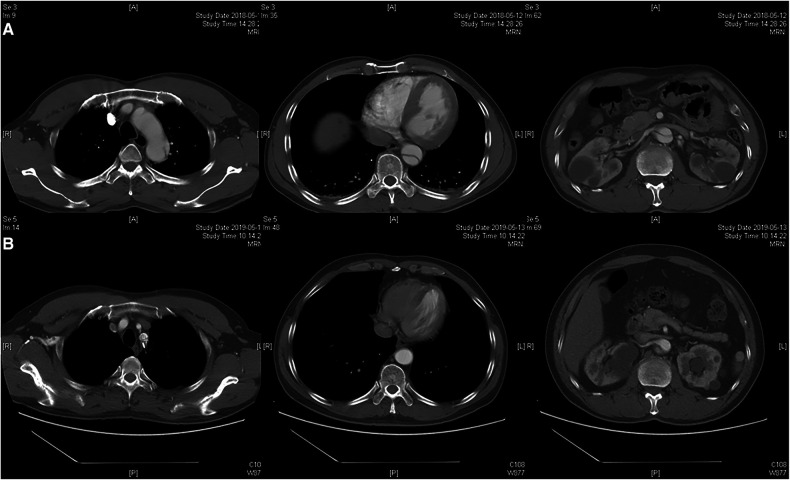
Radiographic image of chimney technique. (A) Preoperative CTA showed the dissection involving the distal aortic arch and visceral aortic segment. (B) Postoperative CTA indicated the patency of the chimney stent and partial thrombosis of the visceral aortic segment during 1-year follow-up.
